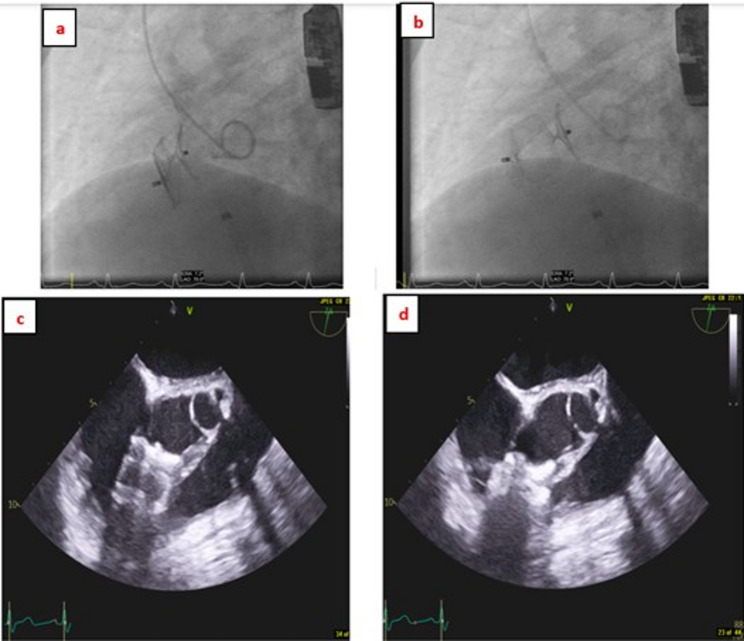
Perimembranous VSD (14 mm) closed using a 22 mm Amplatzer Vascular Plug II (AVP II). The device appeared elongated and demonstrated dynamic compression during systole, with protrusion toward the right ventricular outflow tract (RVOT), resulting in significant ventricular ectopy. a, b Fluoroscopic images showing cyclical shortening of the device during systole. c, d Corresponding transoesophageal echocardiography (TOE) images demonstrating the same dynamic changes. The device was surgically explanted after 9 days, and the VSD was closed successfully. This case highlights the importance of appropriate device sizing and caution when using longer devices in perimembranous VSDs with proximity to the RVOT
